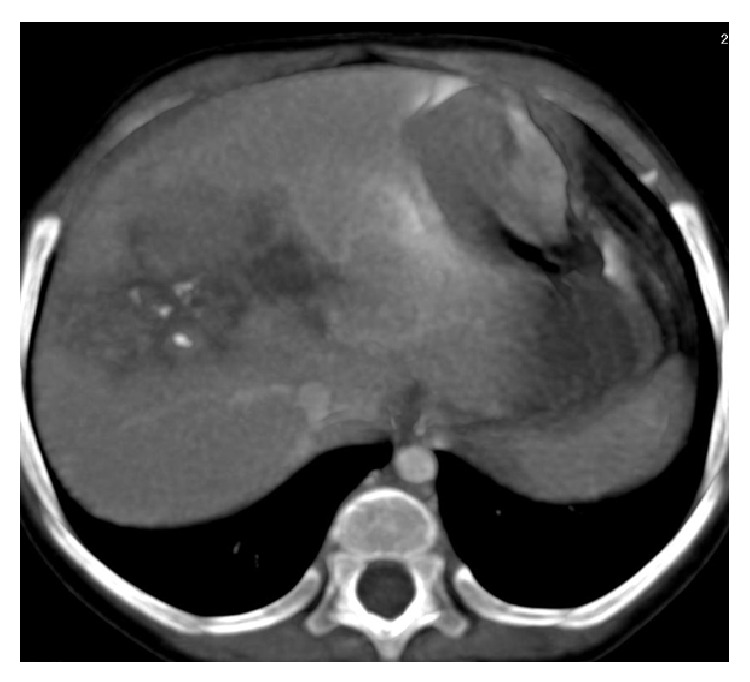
Postchemotherapy CT image shows a heterogeneously enhancing soft tissue density lesion of size 45 × 65 mm, with internal foci of calcification involving right lobe of liver.
